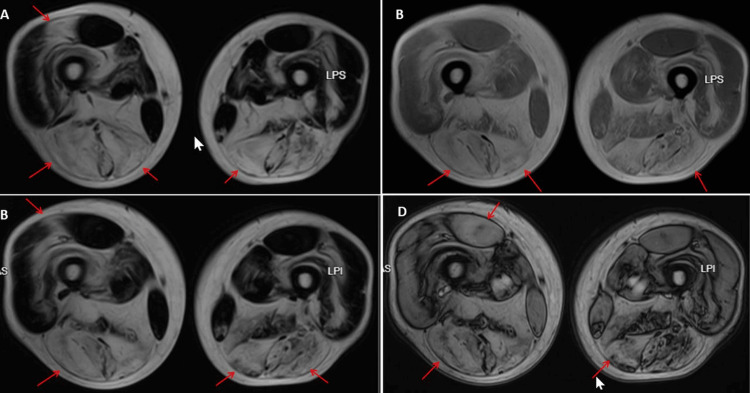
Muscle MRI (T1-weighted imaging, axial view Dixon technique pre in F (A), pre in R (B)) showing hyperintensity (fatty infiltration) in the hamstrings and rectus femoris muscles (arrows), which did not enhance on contrast medium (C, D, arrows).
